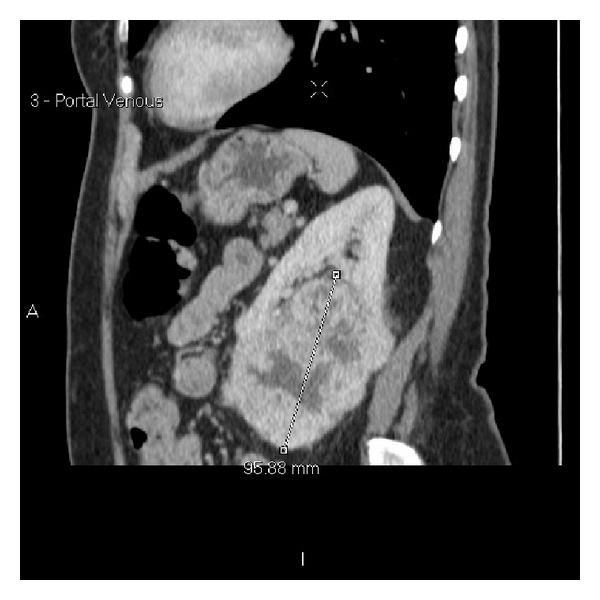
Sagittal portal venous phase staging CT scan demonstrating a 96 mm left renal mass.
